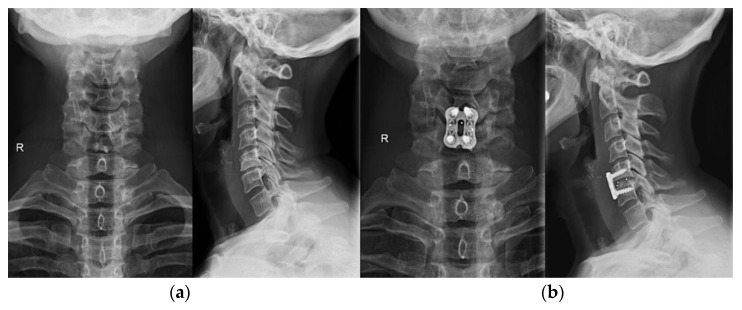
Anterior cervical discectomy and fusion (ACDF). (a) preoperative X-ray; (b) postoperative X-ray of the ACDF (C5–C6 segment).
